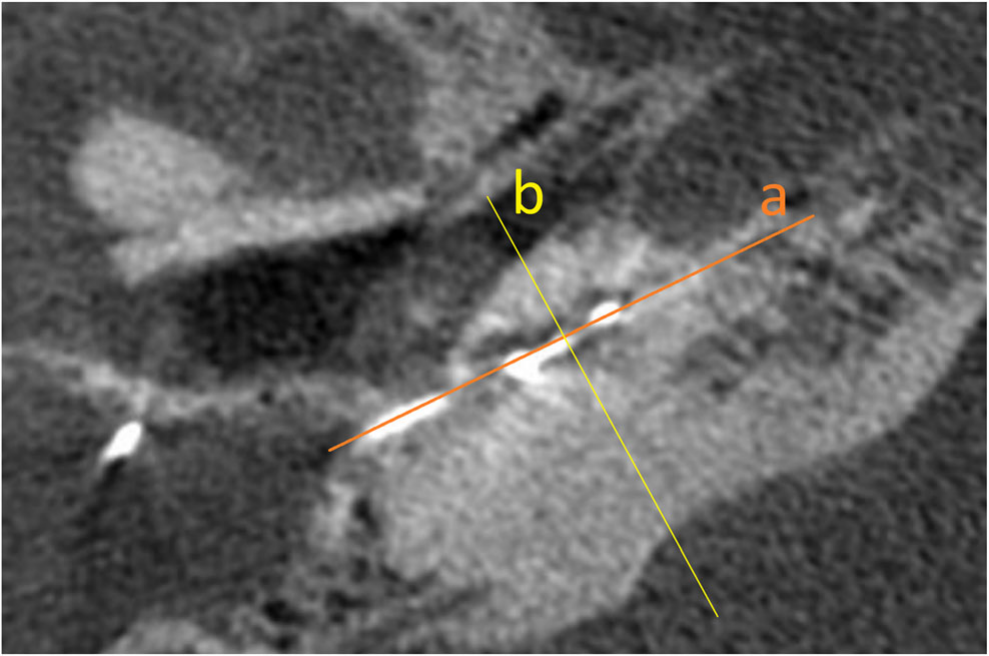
Axial CBCT image shows the reconstruction planes for the different reformatting projections: (a) Cochlear view reformat parallel to the basal turn of the cochlea, (b) Mid-modiolar view reformat perpendicular to the cochlear view plane
